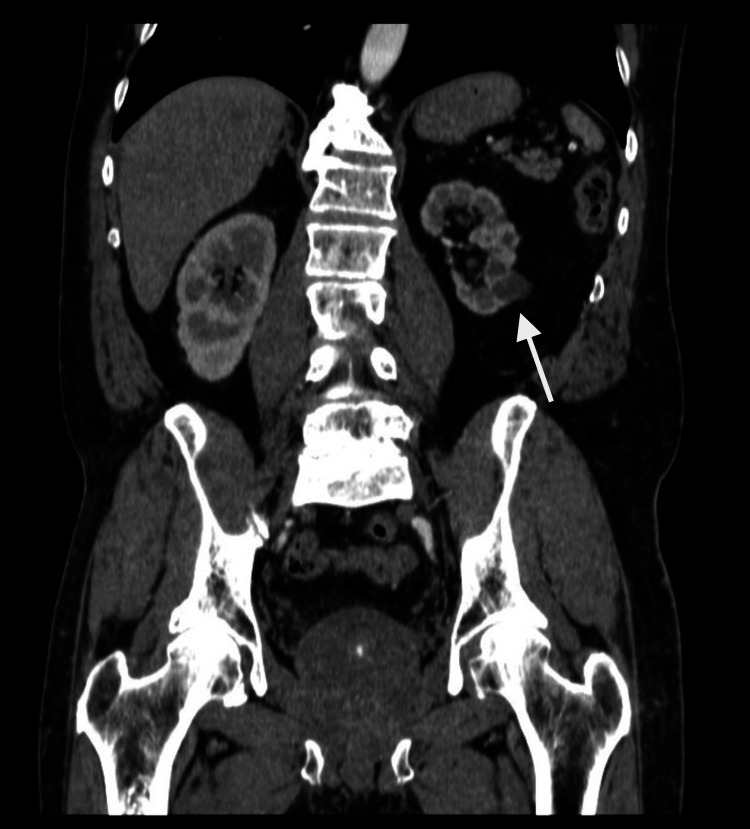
Previous CT scan showing a renal hematoma measuring 1.5 x 1 cm in largest diameter (arrow), located in the lower pole
